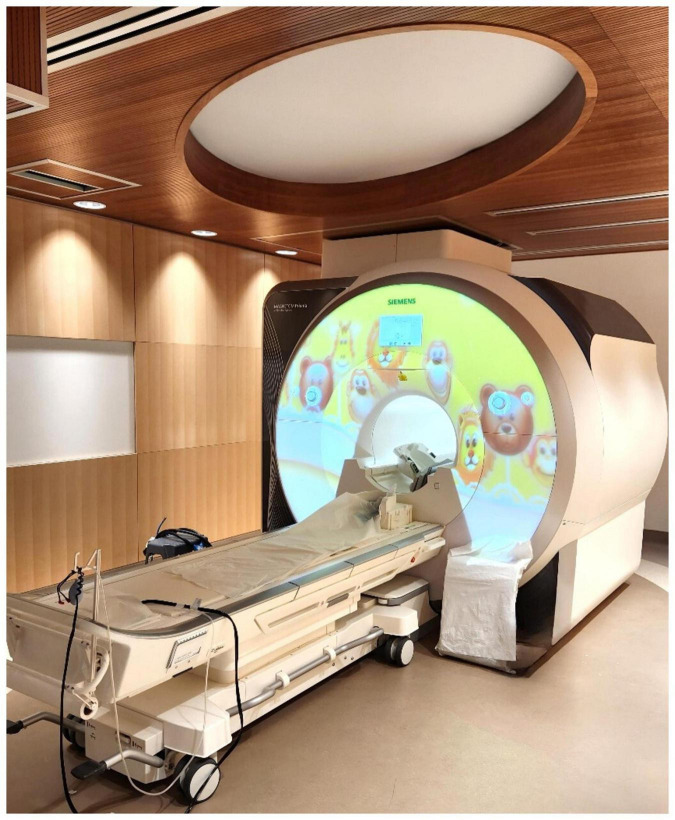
The MRI suite at Holland Bloorview Kids Rehabilitation Hospital (Toronto, Canada). This suite is fully accessible, child-friendly, research-focused, immersive, and customizable. Awake MRI head scanning occurs pre- and post-intervention.
